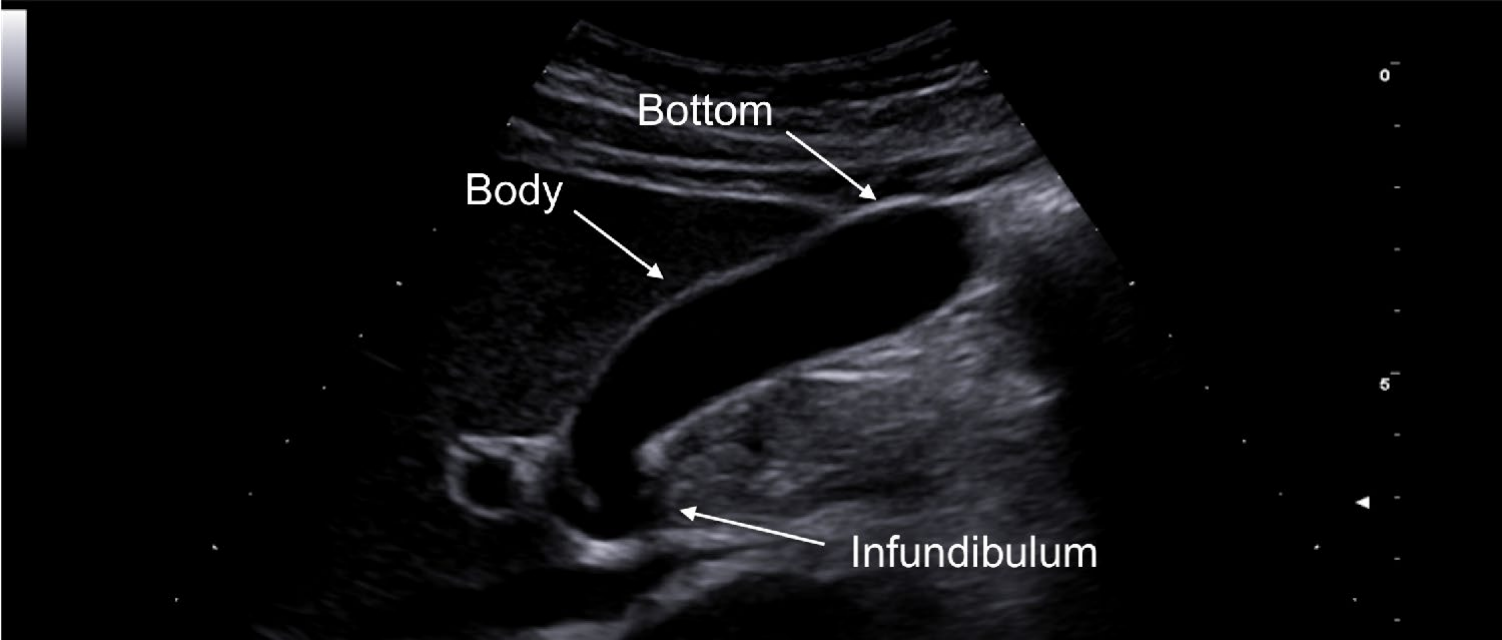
Sonography image depicts a representation of the gallbladder: infundibulum, body, and bottom (white arrows)
